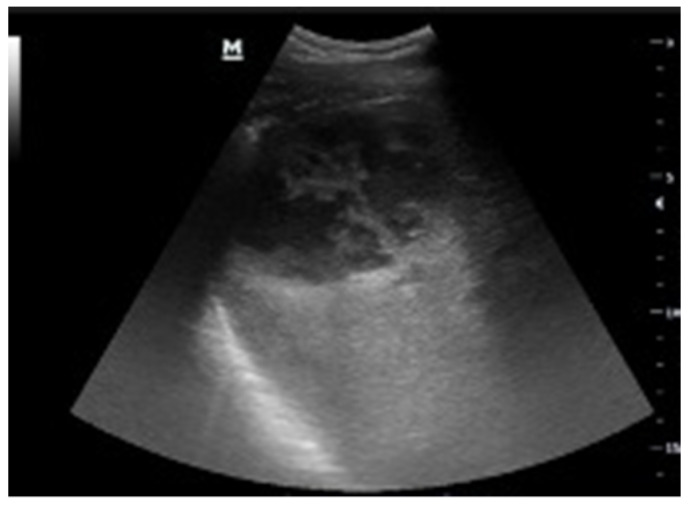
Black where it should not be. A 75-year-old man with recent history of low back pain treated with intramuscular anti-inflammatory therapy. He presented at the emergency department with pain in the left thigh on administration site. The ultrasound showed a corpuscle collection with the typical “swirl sign” during probe compression due to the movement of pus within the fluid collection. Final diagnosis: pyogenic liver abscess.
